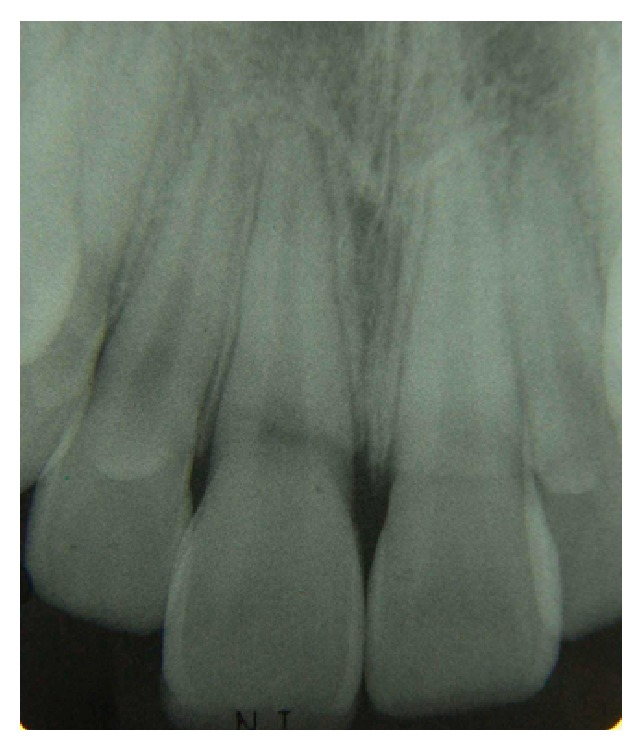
Initial periapical radiograph.
